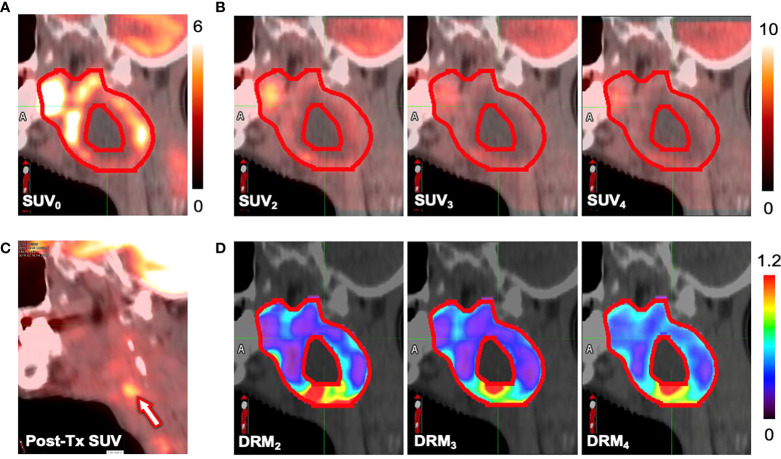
Standard uptake values (SUVs) measured at (A) the pretreatment (SUV_0_), (B) the *i*th treatment week (SUV_i_), and (C) the 6-month posttreatment. (D) The dose–response matrix (DRM) estimated using single feedback image for a patient (primary site: tonsil, stage IV, HPV−) who experienced local recurrence.
